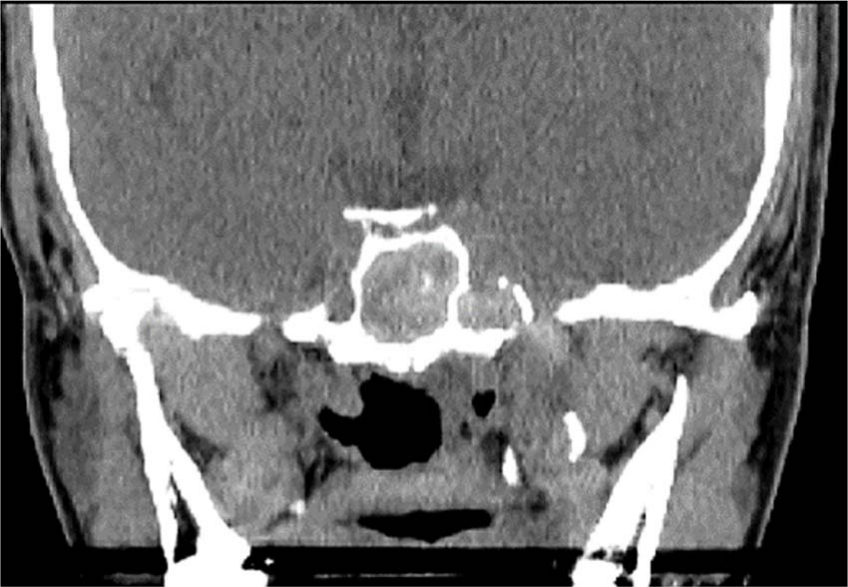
Coronal CT scan image showing complete sphenoid sinus heterogenous opacification.
